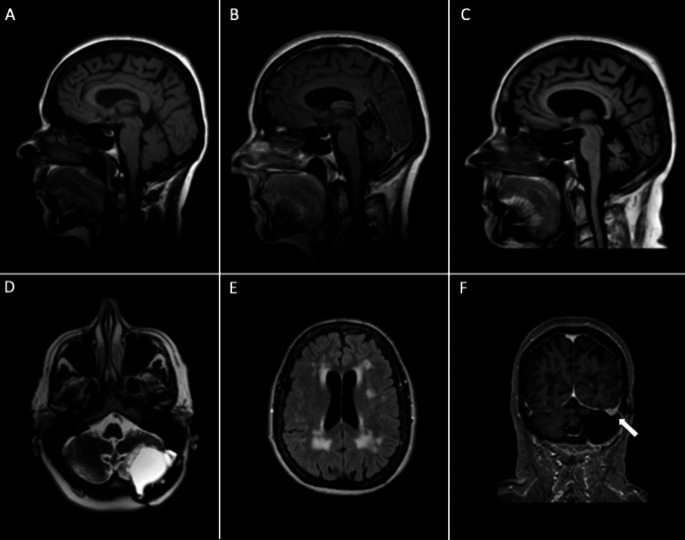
Sagittal T1-weighted brain images showed no cerebellar atrophy at age 54 (one year before surgery) (A); mild cerebellar atrophy, predominantly affecting the anterior lobe and superior part of the posterior lobe of the vermis, was observed approximately 6 months post-surgery (B); by age 71, moderate diffuse cerebellar atrophy was observed (C). Axial T2-weighted image shows the resection cavity on the dorsolateral side of the left cerebellar hemisphere with adjacent gliosis in the left cerebellar hemisphere (D), and confluent supratentorial periventricular white matter abnormalities on T2 FLAIR (E). Coronal T1 after administration of gadolinium contrast shows a small and stable residual meningeoma (F)
